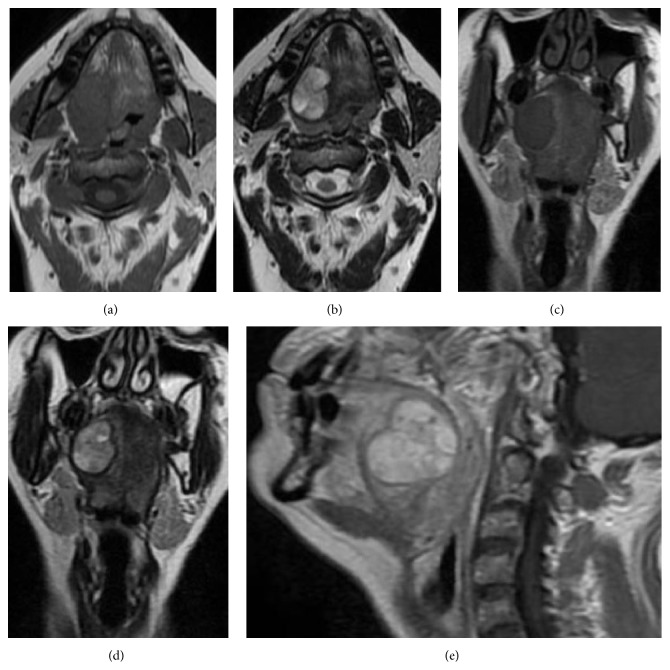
MRI images of axial section ((a-b), T1 and T2 weight) and coronal sections ((c-d), T1 and T2 weight); MRI images of sagittal section (e) showed the enhancing of the tumour mass involving the tongue base after gadolinium administration in T1 weight.
